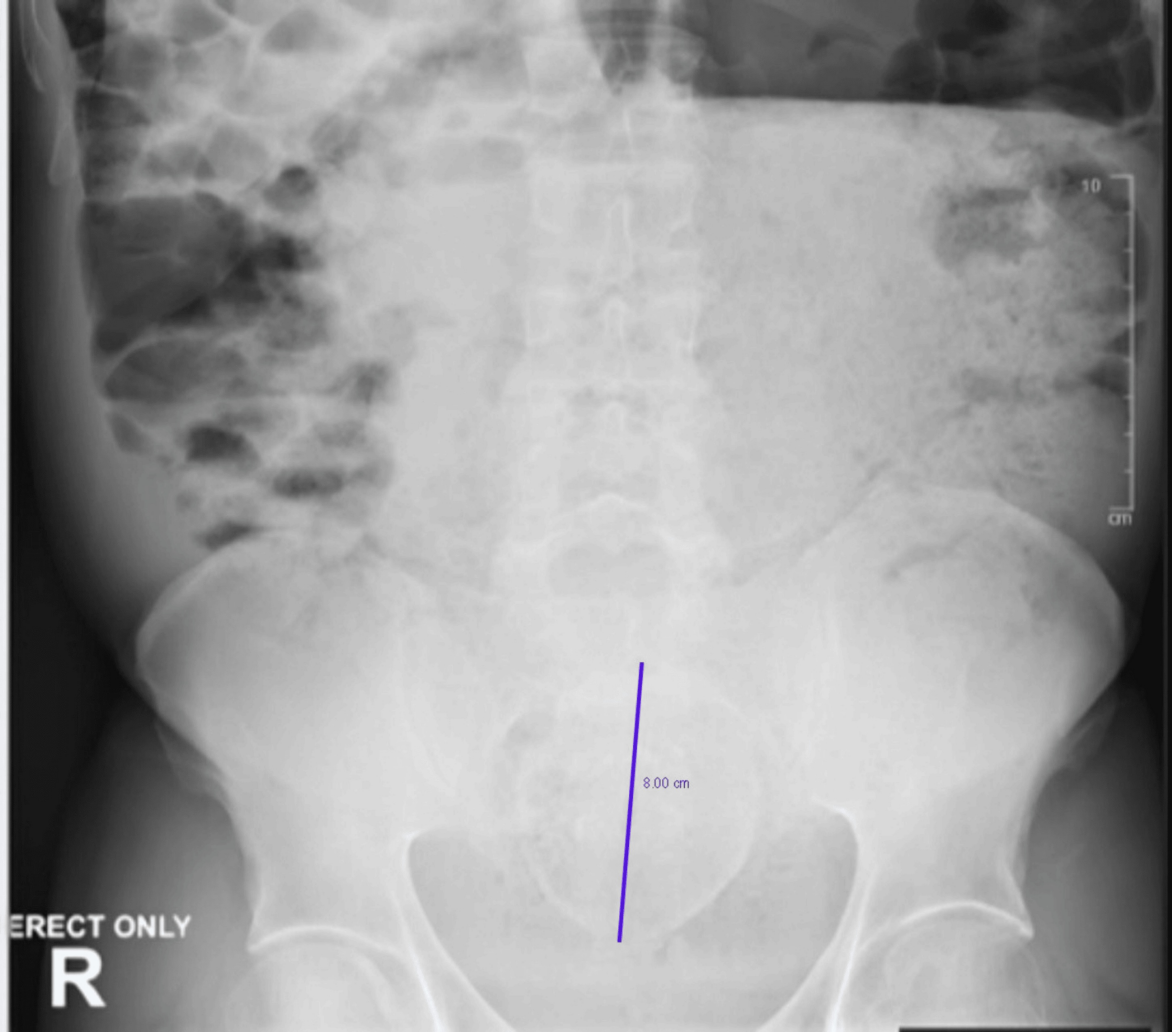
Abdominal X-ray showing a calcified fecalith measuring up to 8 cm in diameter.
